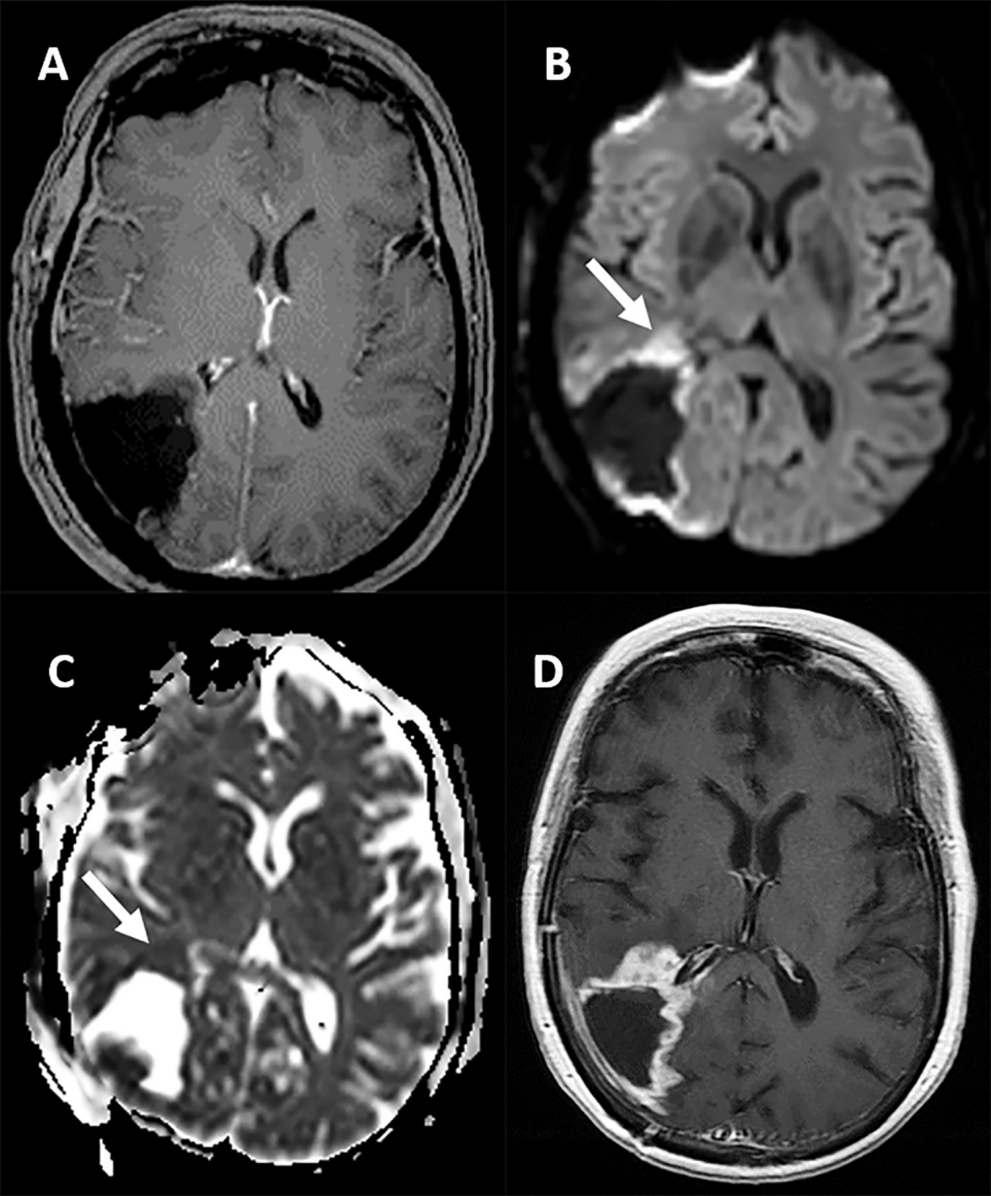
Patient with GBM and a total tumor resection. Patient with GBM and a total tumor resection. A). post-op T1 image with gadolinium demonstrates a cystic cavity without enhancement in the right parietal lobe. There is a rim of restricted diffusion at the margin of the cavity on B) DWI and C) ADC map, representing post-operative change. Note the nodular-like area with restricted diffusion (arrows) anterior to the cavity, which developed a nodular area of tumor progression on the 105 days post-operative MRI (D).
